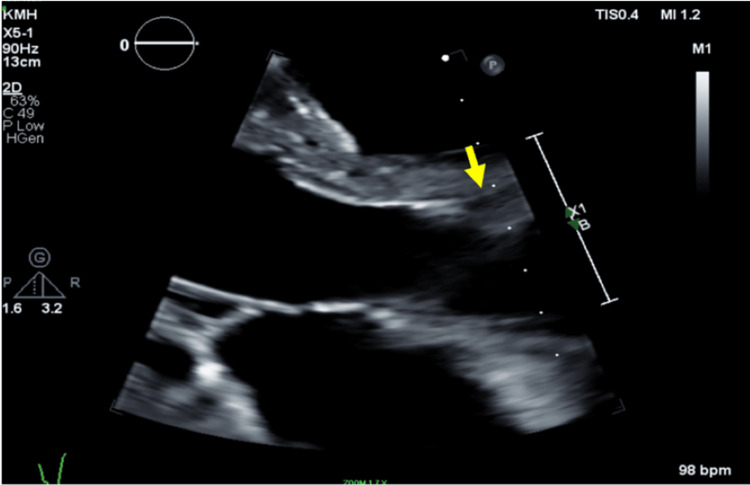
Transthoracic echocardiography (TTE) findings TTE showing parasternal long axis view of the aortic valve and proximal aorta, leaflets are open during systole. Arrow pointing to the right coronary ostium.
